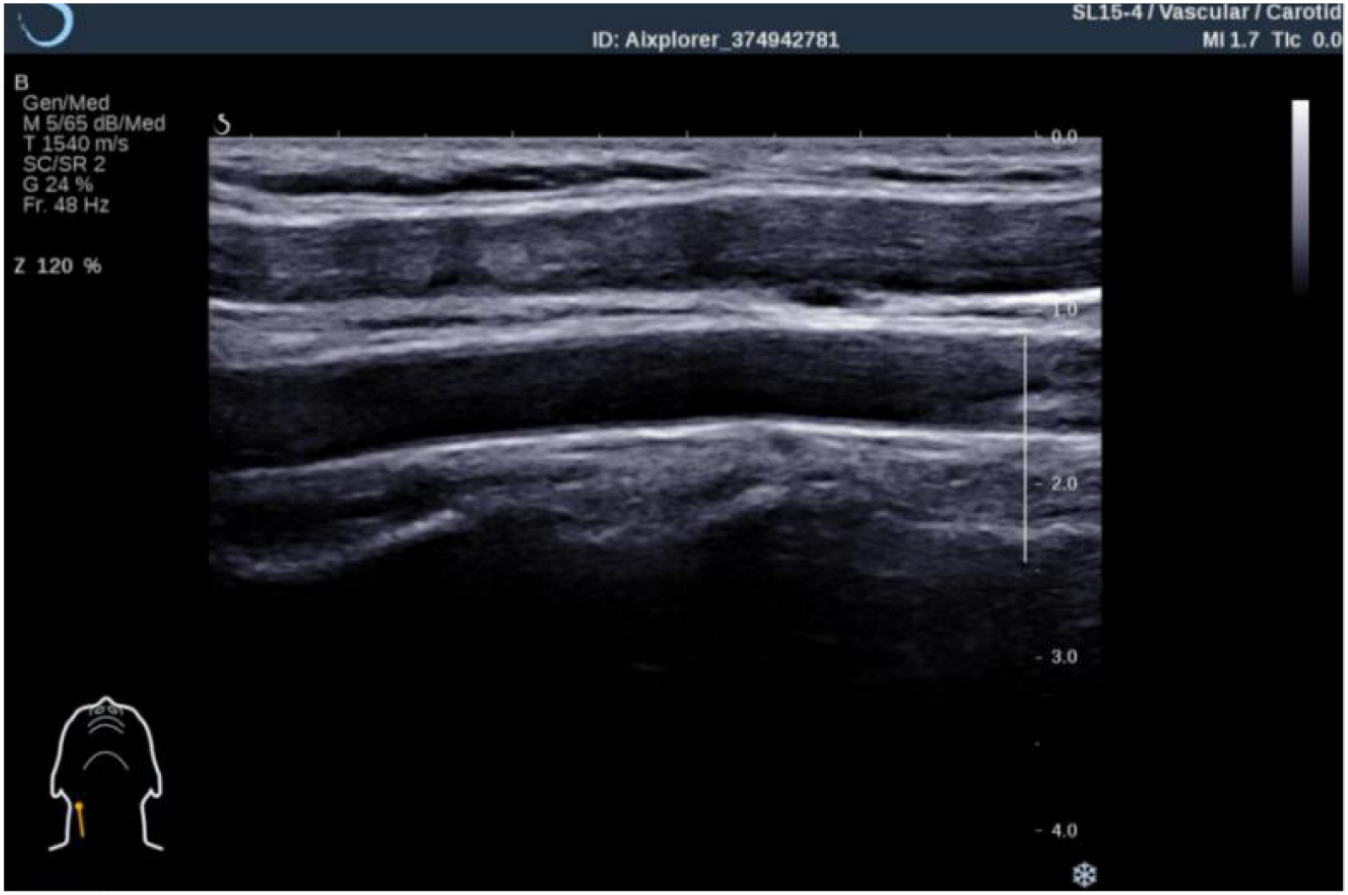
Long-axis image of common carotid artery under two-dimensional gray-scale ultrasound.
